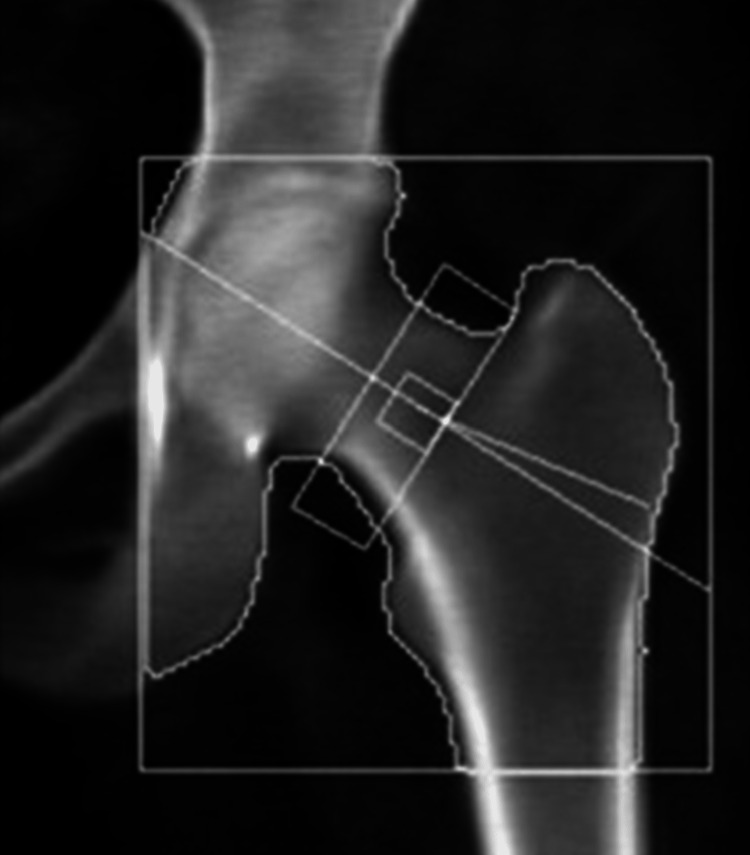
Dual-energy X-ray absorptiometry (DEXA) scan of the femoral neck showing osteopenia
